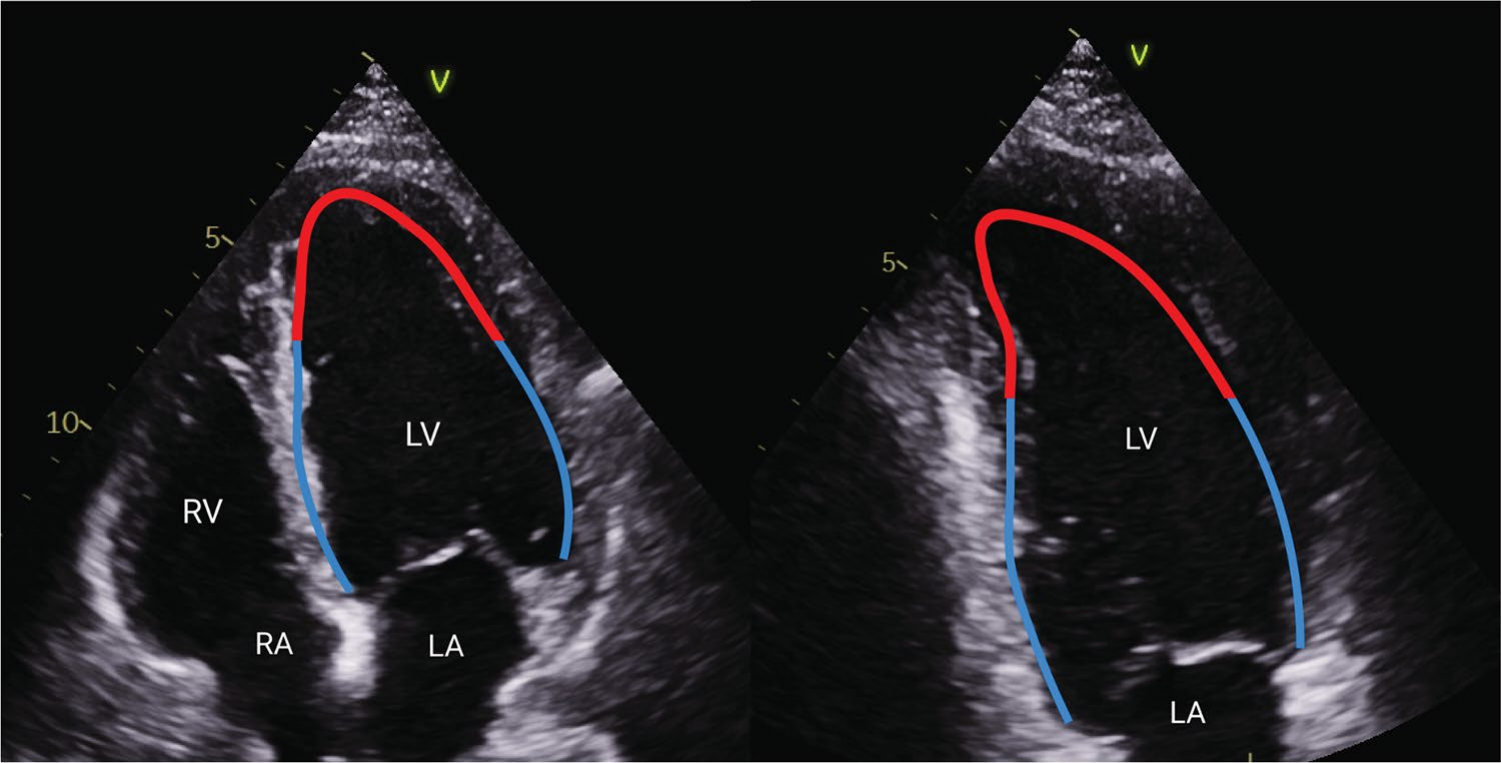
Assessment of the proportion akinesia in the left ventricular four- (left) and two (right) chamber view. First, the left ventricular endocardial border was outlined in apical four chamber view (blue line) in end-diastole. Secondary, the endocardial border and the extent of akinesia was outlined (red line). The total extent of akinesia in millimetres in both the apical four- and two chamber views was divided by the total length in millimetres in both apical four- and two chamber views in diastole multiplied by 100. *RA* right atrium, *RV* right ventricle, *LV* left ventricle, *LA* left atrium
